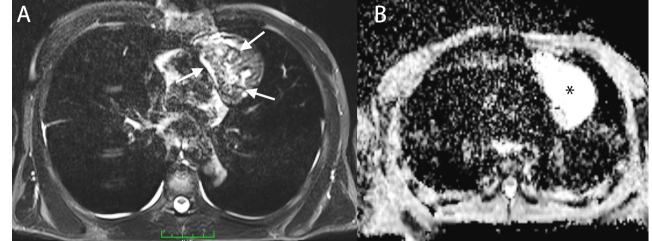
T2-weighted (A) and diffusion-weighted imaging (B) showing a pericardial mass that is heterogeneous isohyperintense with a hypointense wall (arrow). There was no restriction of diffusion on diffusion-weighted imaging (asterisk).
